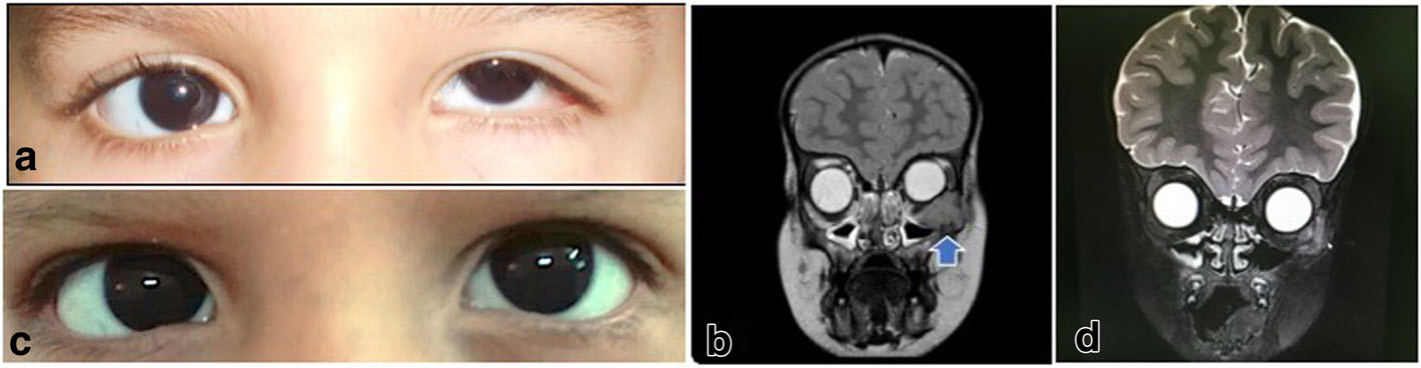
a The clinical appearance of the left displaced globe with narrowing of the palpebral fissure and pseudoptosis. b The large orbital mass lesion along the inferior and lateral orbital bony wall (*arrow head*) on T2-weighted magnetic resonance image at initial presentation. c The appearance of the left eye with resolution of the swelling and the dystopia 9 months after the initiation of chemotherapy. d Repeated T2-weighted magnetic resonance image more than 6 months after treatment with resolved left orbital mass
